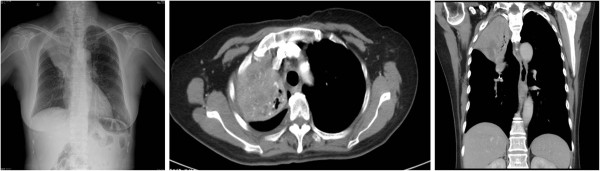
A chest X-ray and CT revealed a space-occupying lesion in the upper lobe of right lung. A chest X-ray and CT revealed a space-occupying lesion in the upper lobe of right lung, which indicated central lung cancer with atelectasis of the right upper lung.
